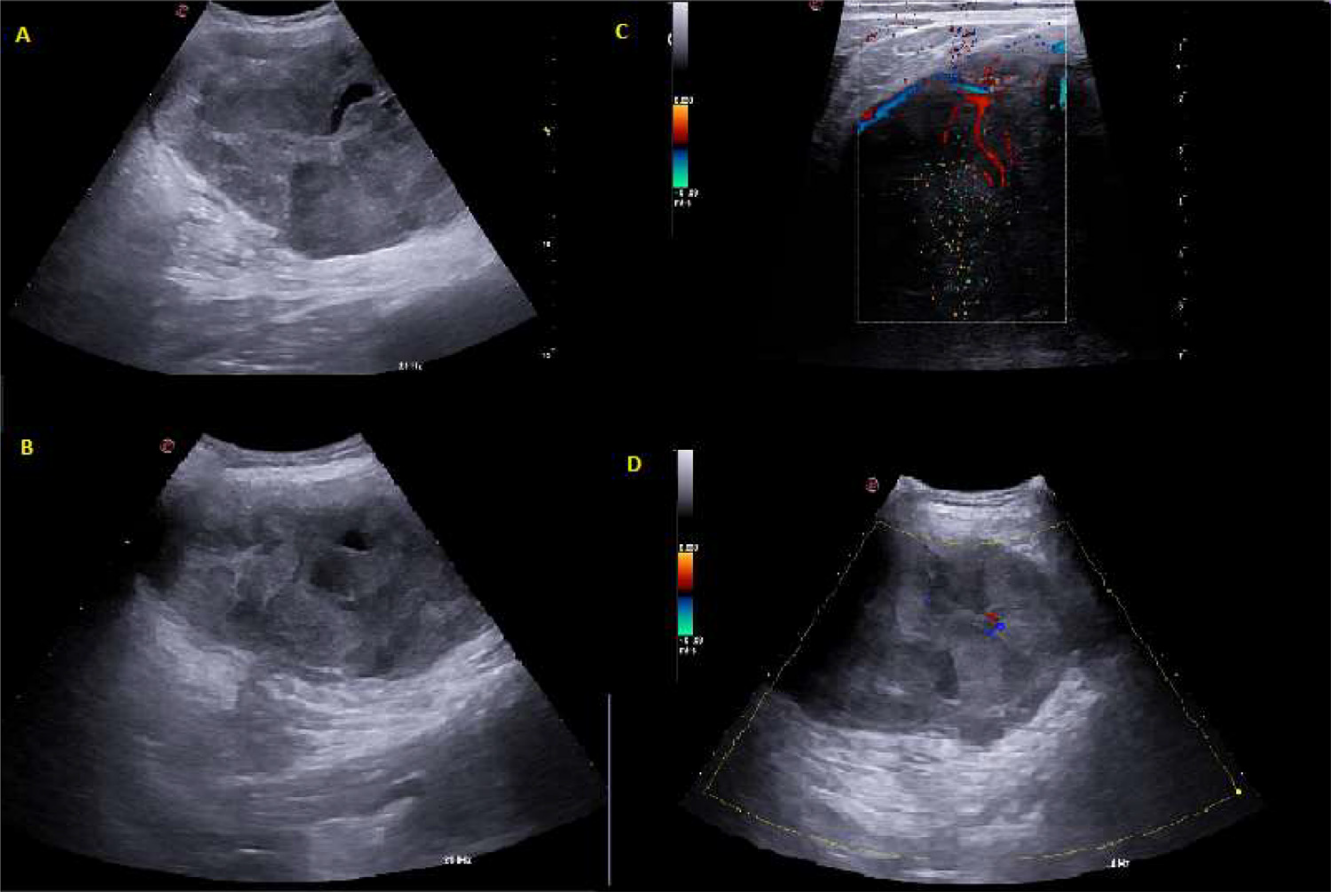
(A and B) Ultrasonography of the formation appears not well characterized given its large extent; it is very inhomogeneous with hypoechogenic/an-echogenic areas mixed with some septa. (C and D) At Colour-Doppler evaluation the vascular signal appears to increase in its vicinity and inside it.
